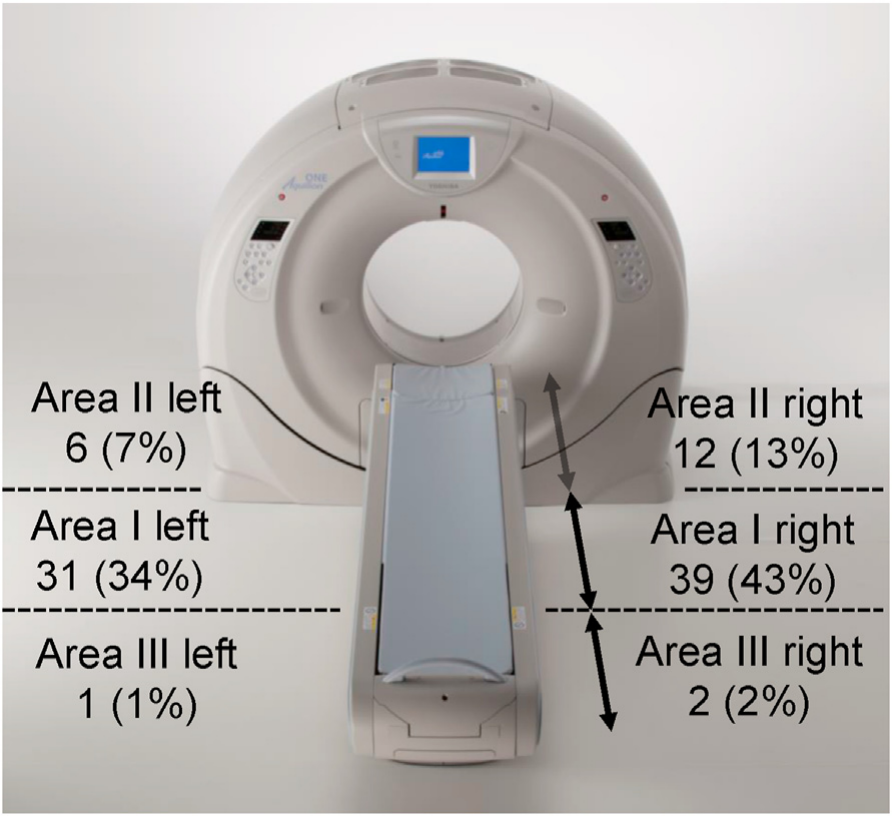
Standing positions of CT-assisting personnel and number of assists (n = 91, June 2017–May 2018). Area I is on the side of the bed near the CT gantry. Area II is on the other side of the bed and near the CT gantry. Area III is on the side of the bed away from the CT gantry. The number of personnel (number of times, %) in each position is listed.
